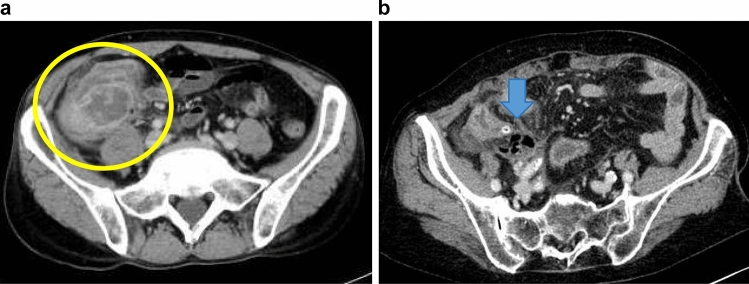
Computed tomography (CT) findings. a Representative case of acute appendicitis with abscess in a patient included in the study. CT image shows a pericecal abscess (yellow circle). b Representative case of acute perforated appendicitis in a patient excluded from the study. CT image shows free gas around the appendix (blue arrow)
